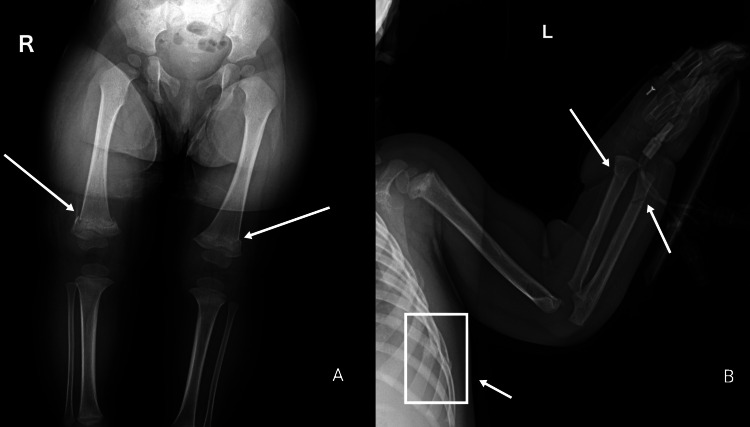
Rib fractures, bilateral classical metaphyseal lesions, and forearm fractures concerning for abuse Multiple fractures as outlined below: The left arm radiograph shows a buckle fracture of the distal radius, oblique fracture through distal ulnar diaphysis (the rib fractures are also visible on this radiograph) The lower limb radiograph shows metaphyseal corner fractures of the distal femurs Case courtesy of Rad_doc, Radiopaedia.org, rID: 47998
